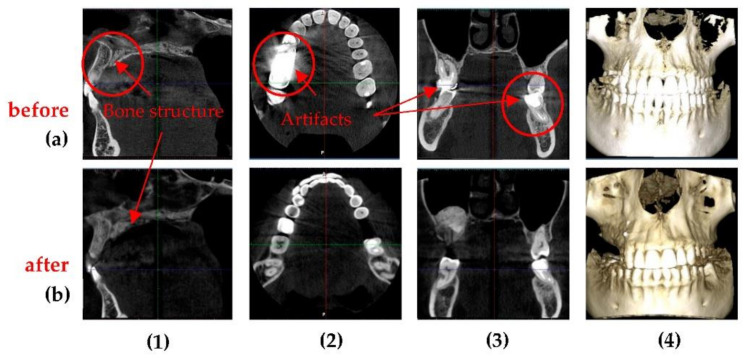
3D CBCT performed on the same patient (F.C., male, 29 years old): images (a) before and (b) after the optimization. Notations: (1) axial, (2) sagittal, (3) coronal, and (4) 3D rendering.
